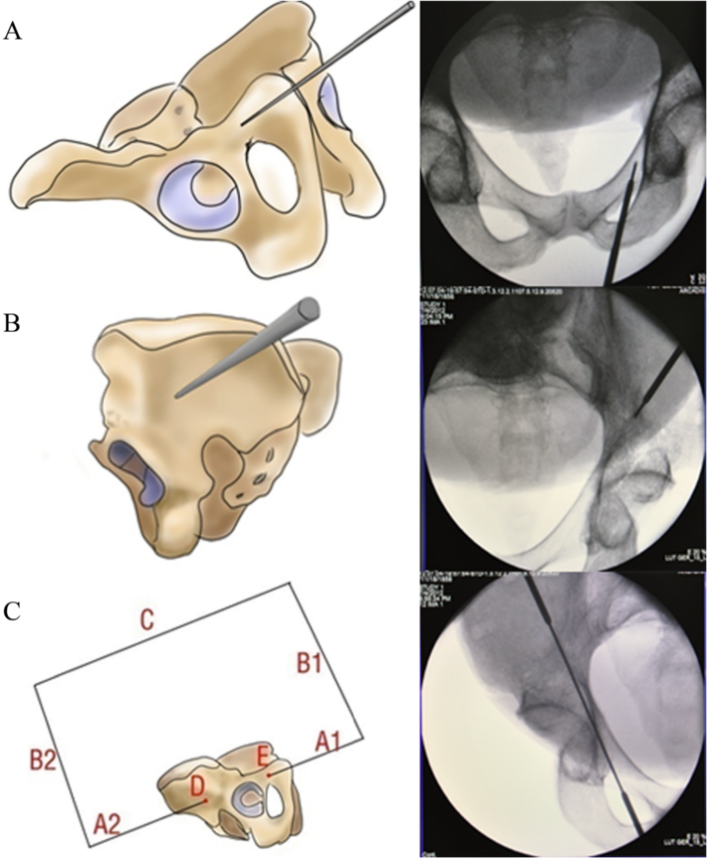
a Locater (A1) is fixed to the obturator crest entry point of acetabular anterior column b Locater (A2) is fixed to the posterior column of ilium outside entry point. c The X-ray of the guide apparatus form the rectangle structure and the guiding needle enters the opposite locater (the guiding needle enters the opposite locater by passing through the obturator crest entry point (E) and the posterior column of the ilium outside the entry point (D))
